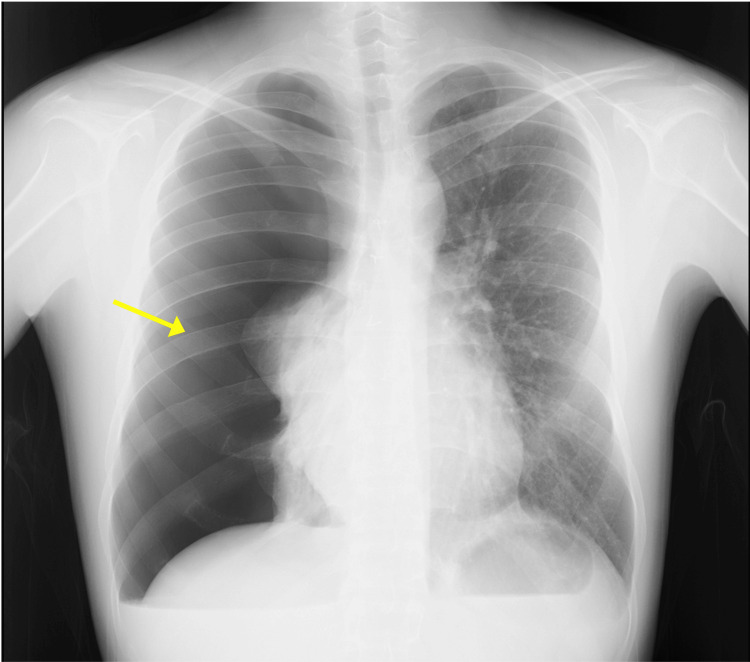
Chest radiograph acquired at the emergency department A massive right pneumothorax (yellow arrow) with a cardiothoracic rate of 41.9% is observed in the upright position.
